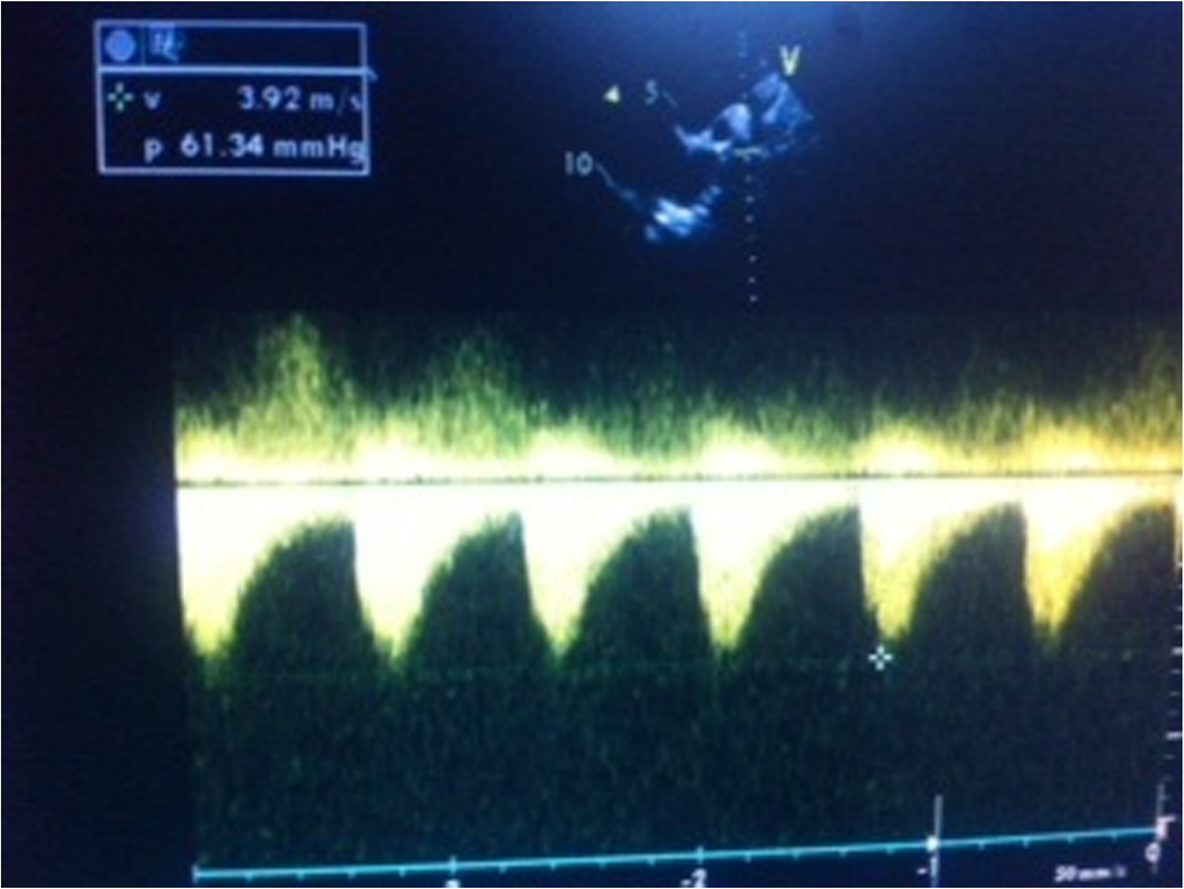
Echocardiography supra sternal view shows aortic coarctation with dilation of the aortic segment proximal to the stenosis and acceleration of the flow.
